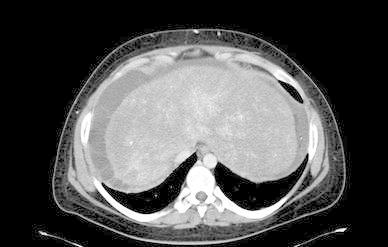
Abdominal CT scan showing inhomogeneous liver parenchymal enhancement after i.v. contrast injection, patency of the inferior vena cava with lack of visualization of the hepatic veins
